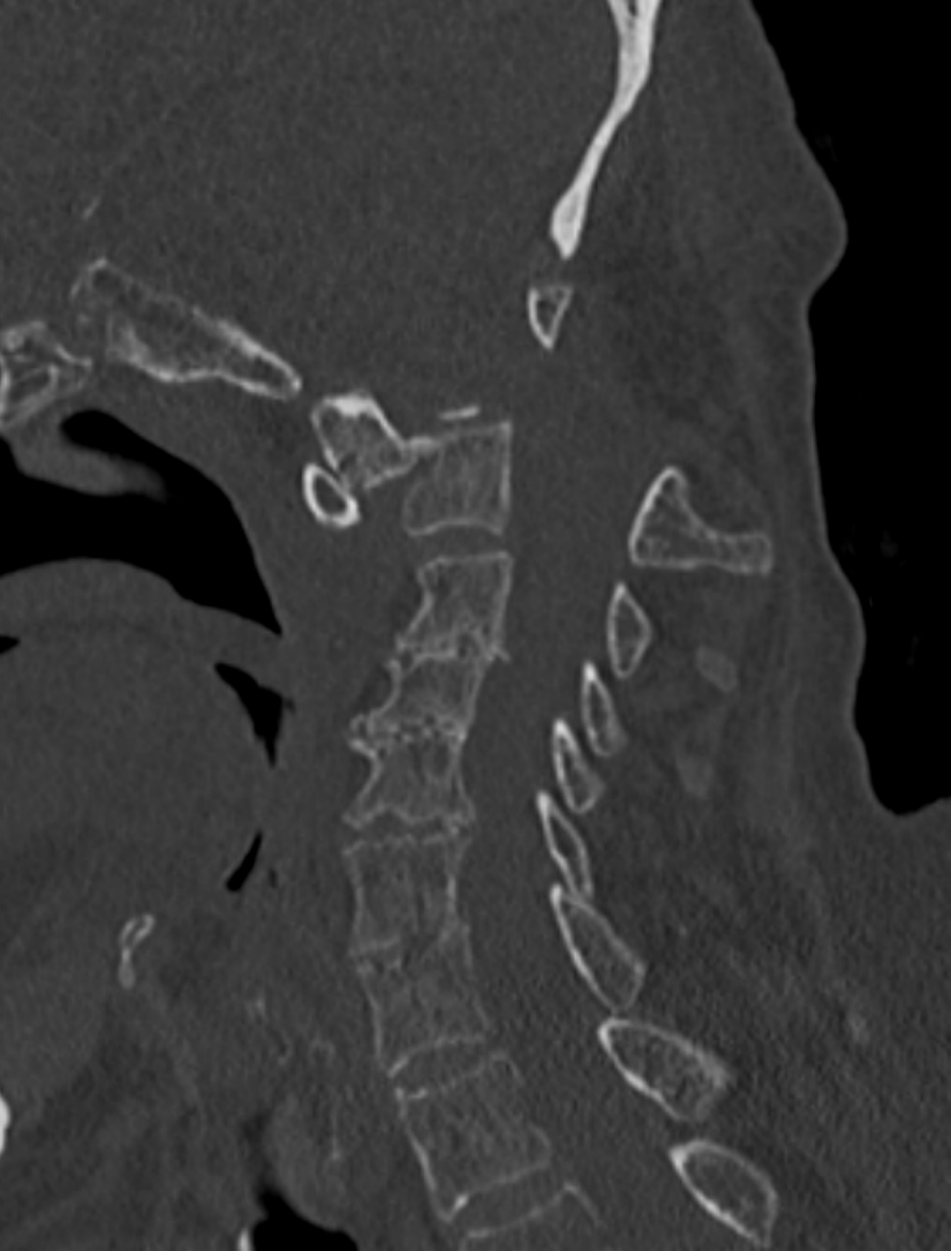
Computed tomography scan of the cervical spine revealed nonunion of an odontoid fracture with C1–two subluxation.
